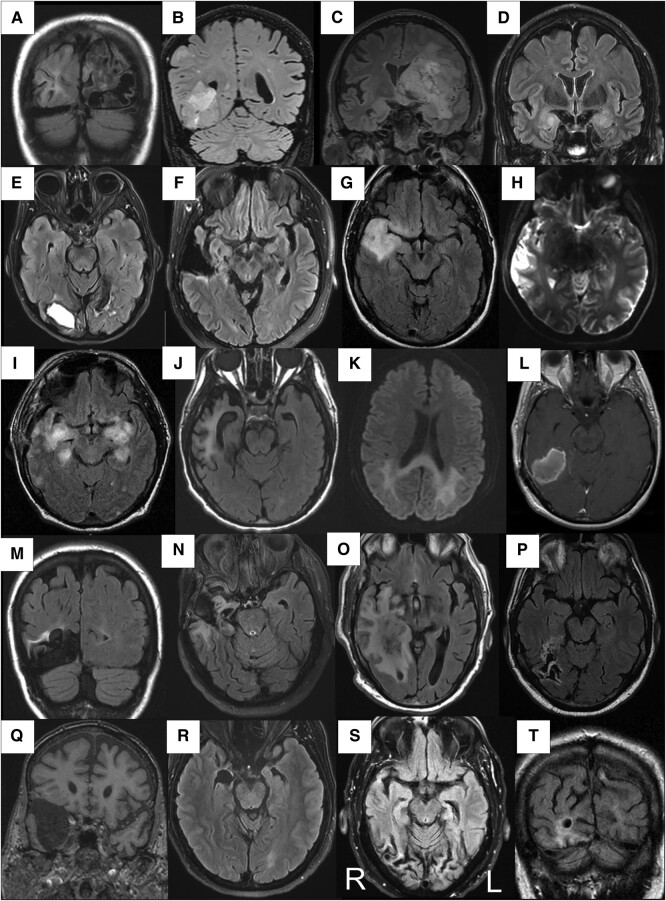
MRI scans of structural abnormalities in patients with a non-degenerative diagnosis. This figure shows a wide range of diverse types of lesions identified on head MRI scan in the patients with non-degenerative prosopagnosia. R, right hemisphere; L, left hemisphere. All images are fluid-attenuated inversion recovery (FLAIR) sequences except for images H and K (diffusion-weighted images), L (T1 + gadolinium) and Q (3D fobl). Epilepsy-related lesions associated with prosopagnosia are shown in panels A, F and M (area of encephalomalacia). Tumour-related lesions associated with prosopagnosia are shown in panels B and C (glioblastoma multiforme), G and L (oligodendroglioma) and O. Other lesion-related prosopagnosias are shown in D (limbic encephalitis), E (stroke), H (mitochondrial encephalopathy, lactic acidosis and stroke-like episodes), I (necrotizing meningoencephalitis), J (post-herpetic encephalomalacia), K (toxic encephalopathy), N (right temporal lesion), O (mass of undermined aetiology), P (post-stroke encephalomalacia), Q (temporal lobe cyst), R and S (post-traumatic encephalomalacia) and T (post-traumatic contusion). All panels show lesions affecting either the right temporal lobe or the right occipital lobe except for C that shows an extensive infiltrative glioblastoma multiforme located primarily in the left hemisphere. All images shown are from adult except for A, which is from an adult patient with developmental prosopagnosia, whose MRI scan at age 7 is shown.
